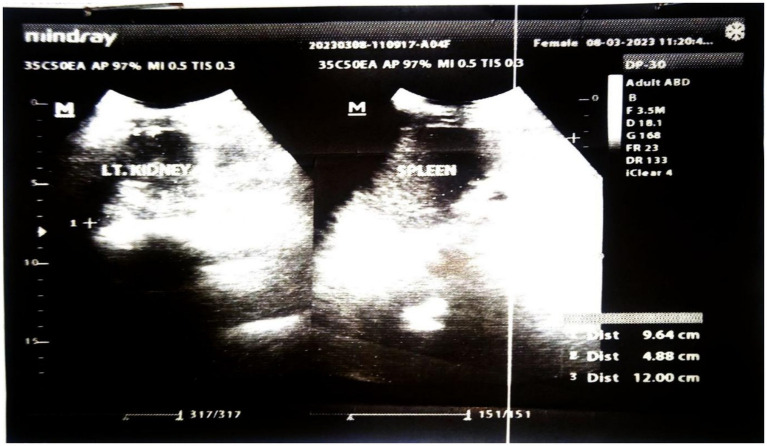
An abdominal ultrasound showing normal-sized kidneys with echogenic cortices and a loss of corticomedullary differentiation (August 2023).
